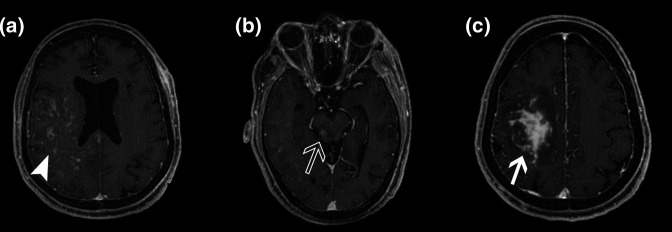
(a) The axial view of contrast MRI scan of the brain showing multiple contrast enhancing lesions involving the right cerebral hemisphere (white arrowhead). (b) The axial view of contrast MRI scan of the brain showing contrast enhancing lesion in the brainstem (empty arrow). (c) The axial view of contrast MRI scan of the brain showing right frontoparietal white matter contrast enhancing lesion (white arrow).
